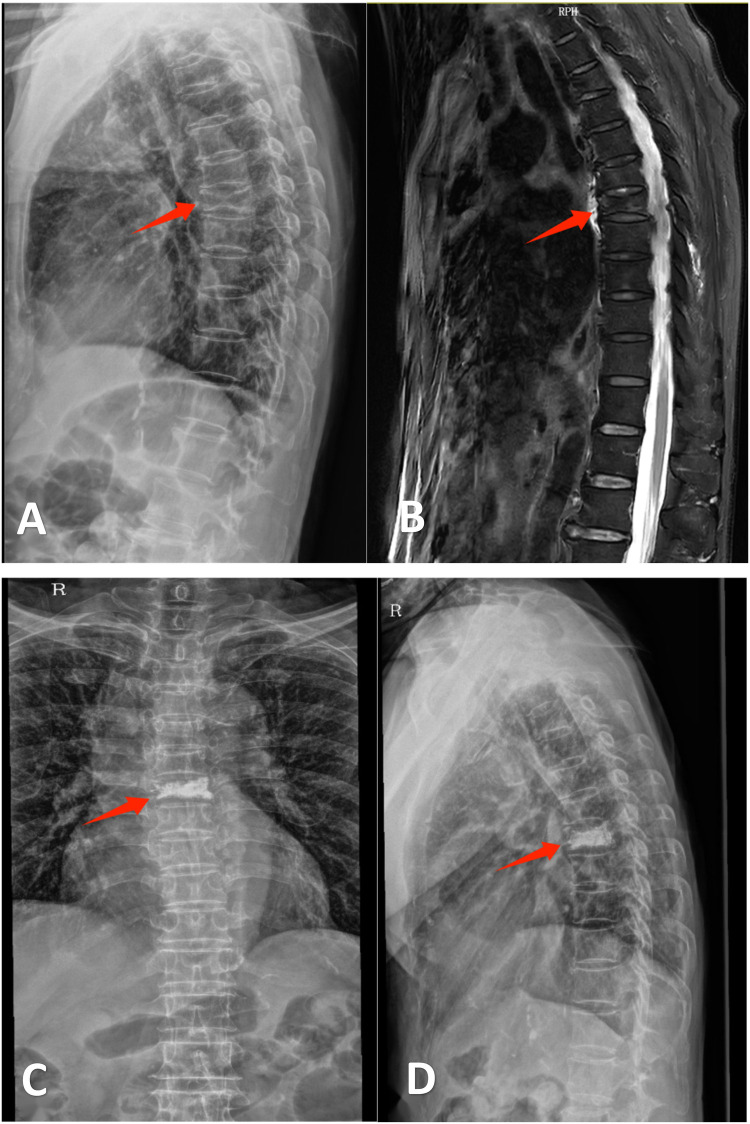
Ms. Zhou, a 76-year-old female, was admitted for “thoracic back pain persisting for 4 days following a twisting injury.” Diagnosis: T6 vertebral compression fracture. She underwent unilateral percutaneous kyphoplasty (PKP). Postoperative pain was significantly alleviated, thus permitting same-day discharge. (A) Lateral radiograph demonstrating wedging deformity of the T6 vertebral body. (B) Sagittal MRI revealing a T6 vertebral fracture. (C,D) Postoperative anteroposterior and lateral radiographs after unilateral PKP at T6.
